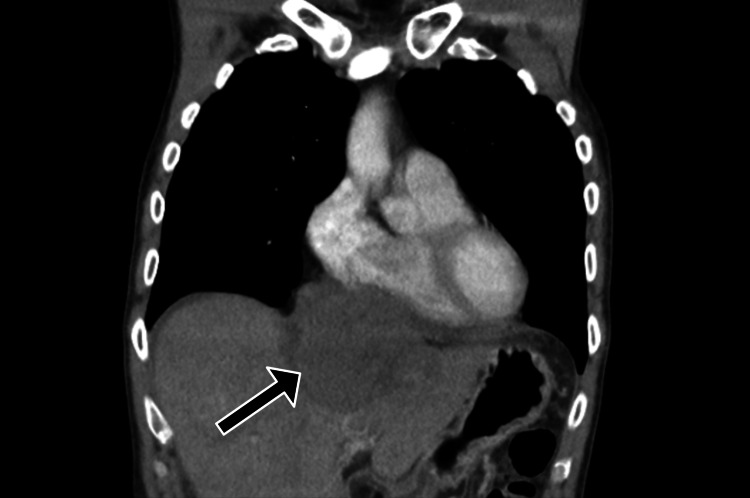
Coronal chest slice from a portal venous phase CT demonstrating a large epigastric mass with local invasion into the liver and pericardial thickening, raising concern for possible pericardial involvement.
